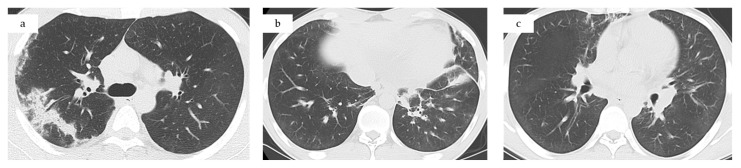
Chest CT at (a) initial presentation, (b) relapse of chronic eosinophilic pneumonia, and (c) current admission, all showing bilateral ground-glass opacities and patchy consolidations.
